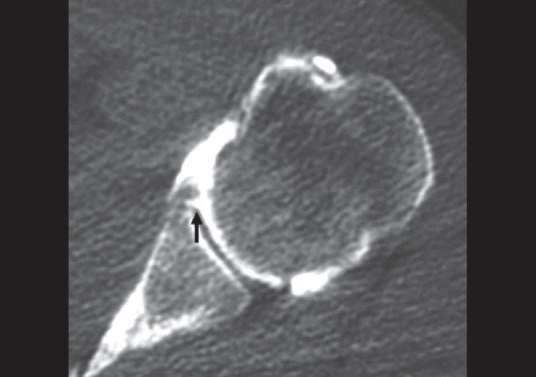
Axial CT-Arthrography image of a 40-year-old man wit arthroscopy proved GLAD lesion. Note the presence of contrast media in the glenoid cartilage (*arrow*)
